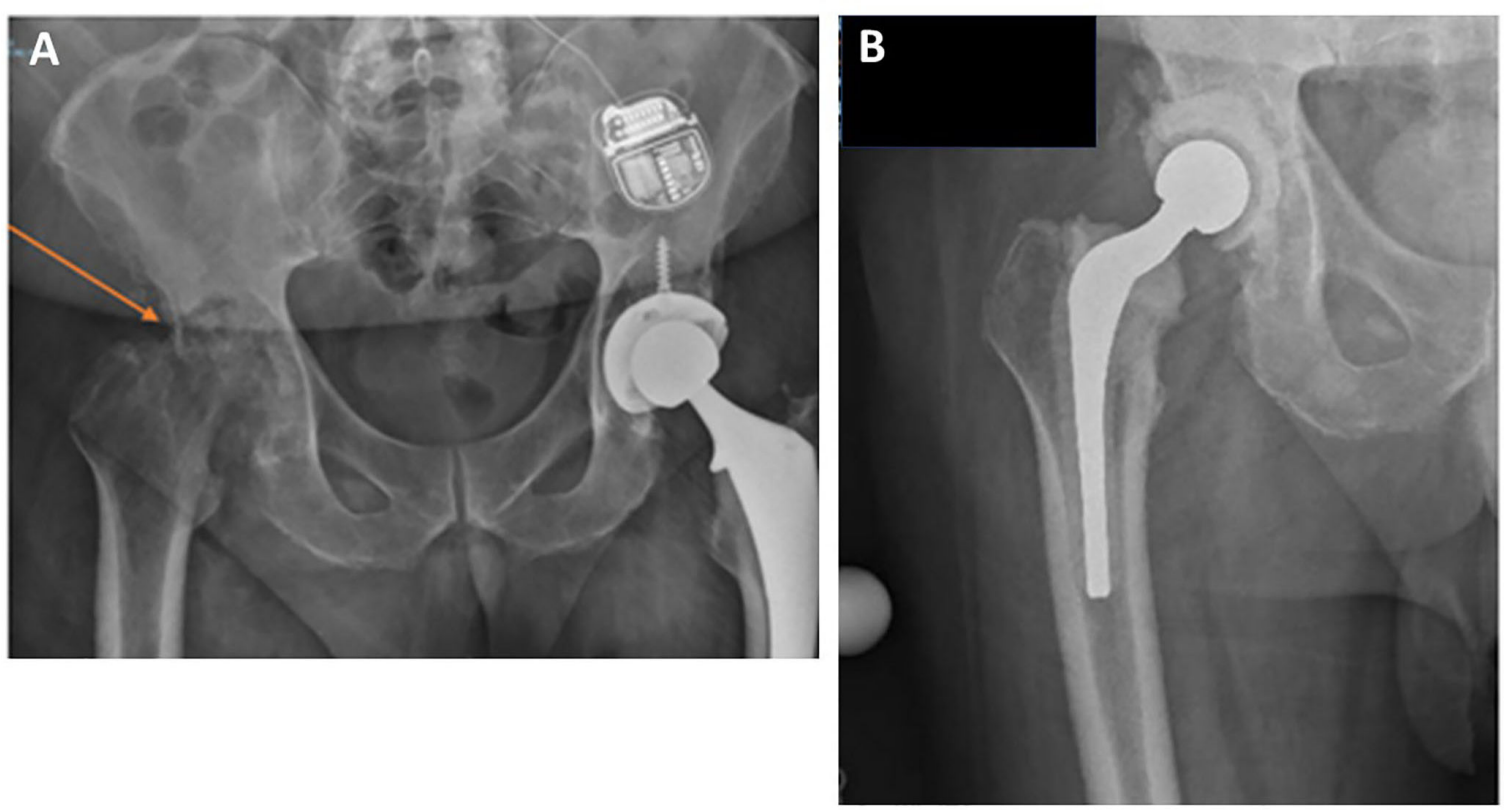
(A) X-ray image of the right hip of a patient with destructive changes of his hip (see arrow) and (B) X-ray image of the right hip of the patient post-surgery done to replace his native hip with an antibiotic spacer.
